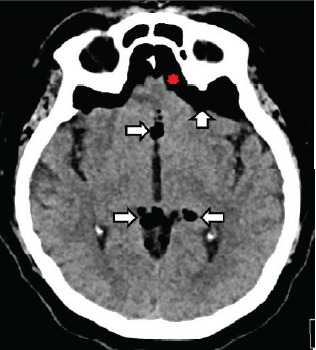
Axial image of non-contrast computed tomography of the head depicting intracranial air (white arrows). The red asterisk indicates the Mount Fiji sign, which is defined by frontal lobe separation due to subdural air.
